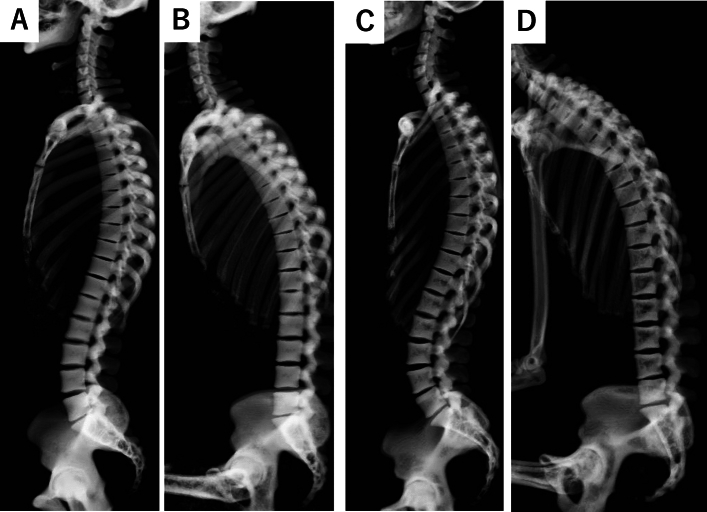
Representative cases of the change of spinal alignment by posture in the young subjects. (A) The standing whole spine CT of 32-year-old male. (B) The sitting whole spine CT of 32-year-old male. (C) The standing whole spine CT of 38-year-old female. (D) The sitting whole spine CT of 38-year-old female. A significant spinal alignment change was observed in a female patient when transitioning to a sitting position.
